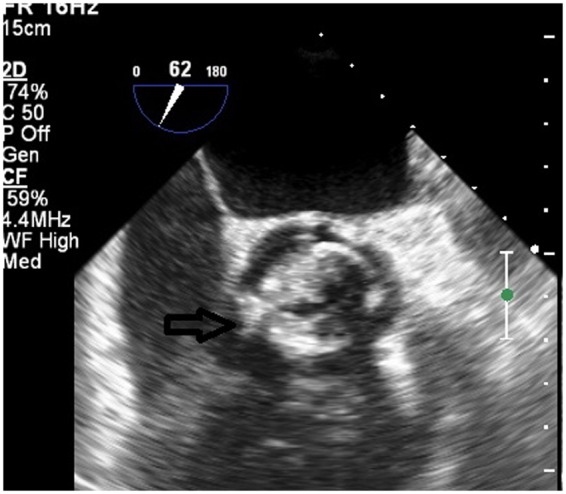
Transoesophageal echocardiogram mid-oesophageal short-axis view showing bioprosthetic aortic valve with severe stenosis and calcification (arrow pointing).
